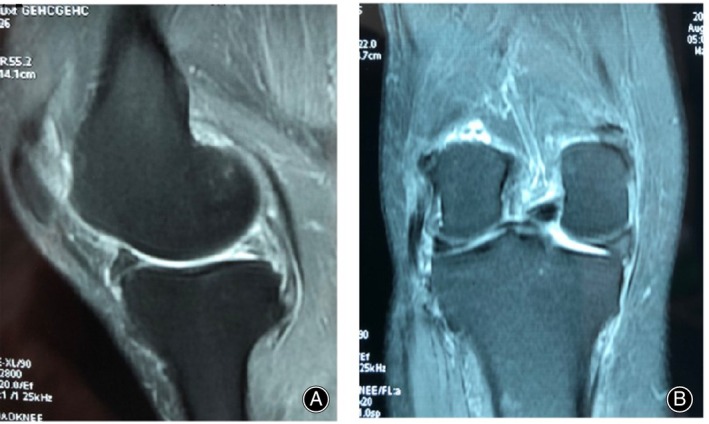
Preoperative magnetic resonance imaging (MRI) of the affected knee joint. (A) sagittal scans; (B) coronal scans.
